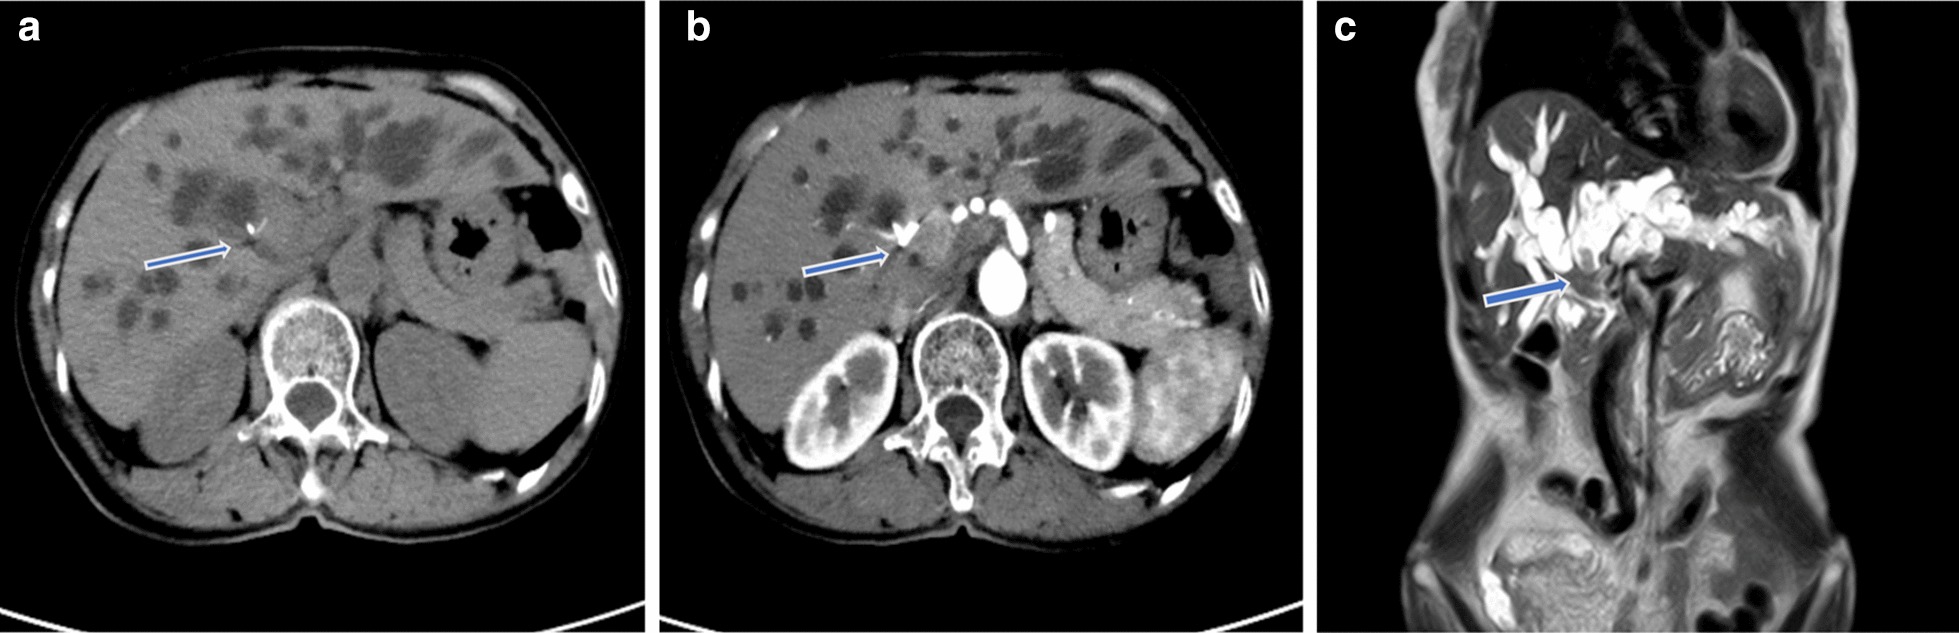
Preoperative imaging findings showed hilar bile duct mass. a CT scan showed that the liver tissue area saw soft tissue-like density lesions, and the bile ducts above the lesions were significantly expanded. b CT enhanced scan indicates that the lesion is mildly enhanced. c MRCP showed truncation of the hilar bile duct, and the intrahepatic bile duct was significantly dilated above the obstruction
